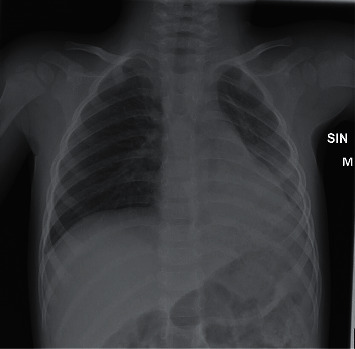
Chest X-ray at arrival, showing infiltrates and/or atelectasis in the left lower lobe.
